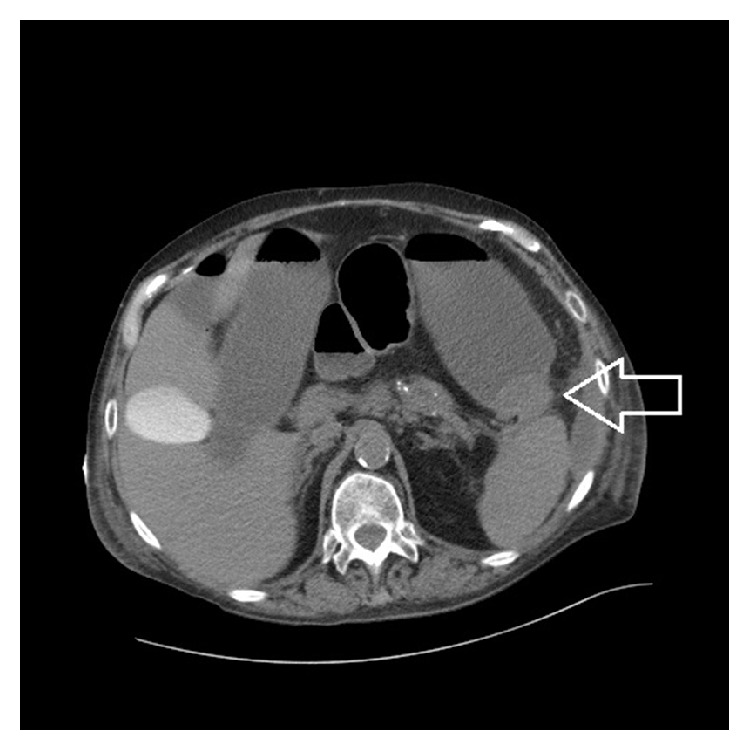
CT abdomen/pelvis done at admission to tertiary medical center demonstrating cut-off sign at the splenic flexure with distended transverse colon.
